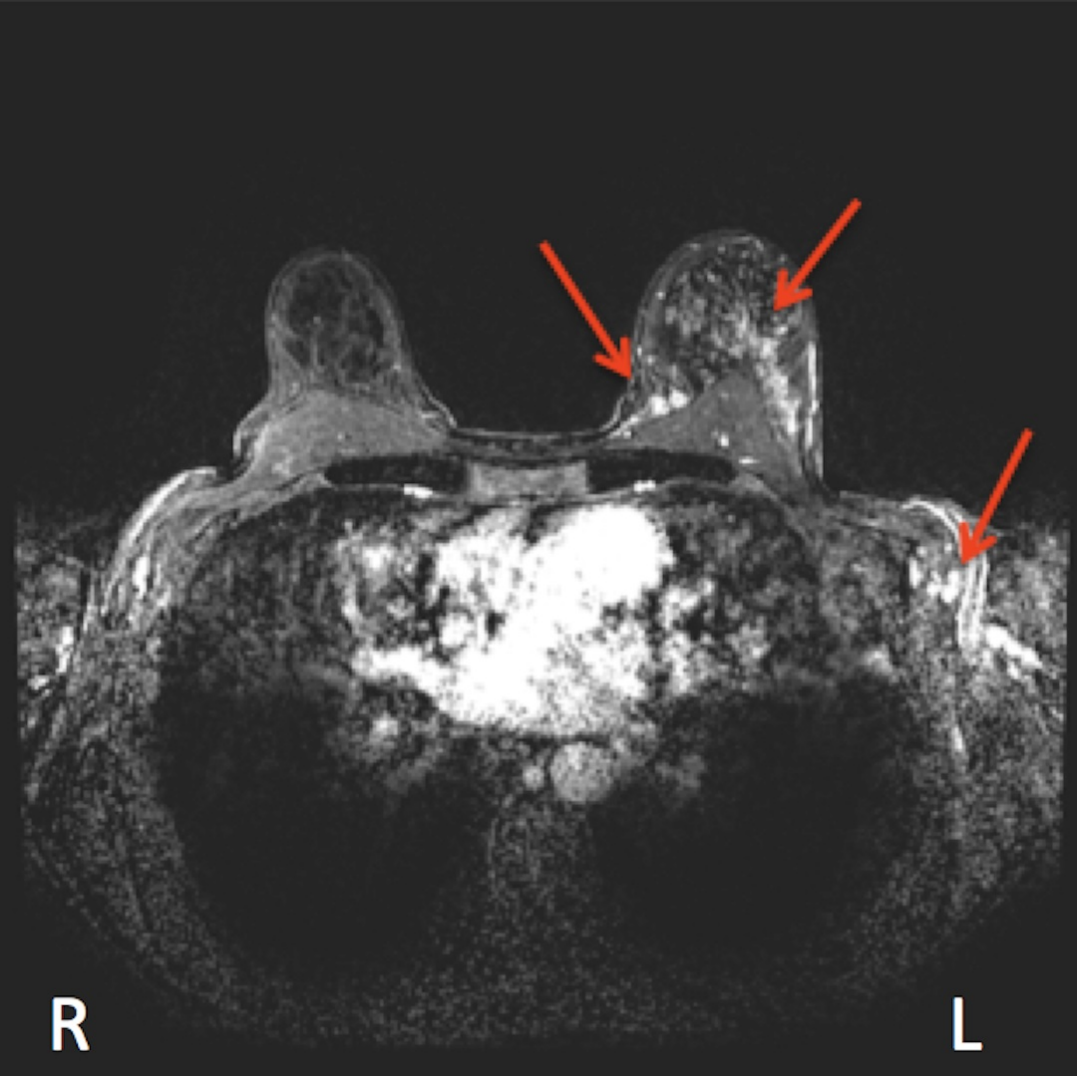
Magnetic resonance imaging before treatment; red arrows indicate multifocal tumors within the breast and multiple left axillary lymphadenopathy
